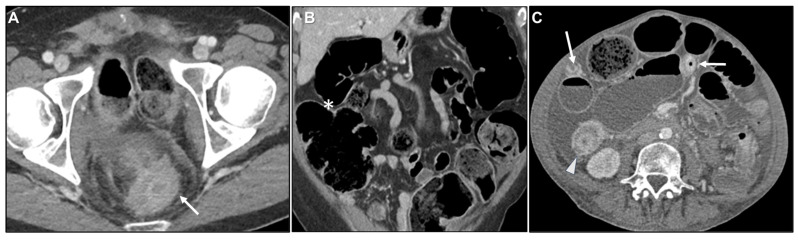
Bowel obstruction. (A) Axial contrast-enhanced CT (CE-CT). (B) MPR CE-CT. Patient with bladder adenocarcinoma with linitis plastica-like rectal metastasis (arrow in (A)) presenting as a colonic obstruction (* in (B)). (C) Axial CE-CT. Patient with linitis plastica-like metastases from invasive lobular carcinoma to small bowel (arrows) and ascending colon (arrowhead) resulting in obstruction at multiple levels.
